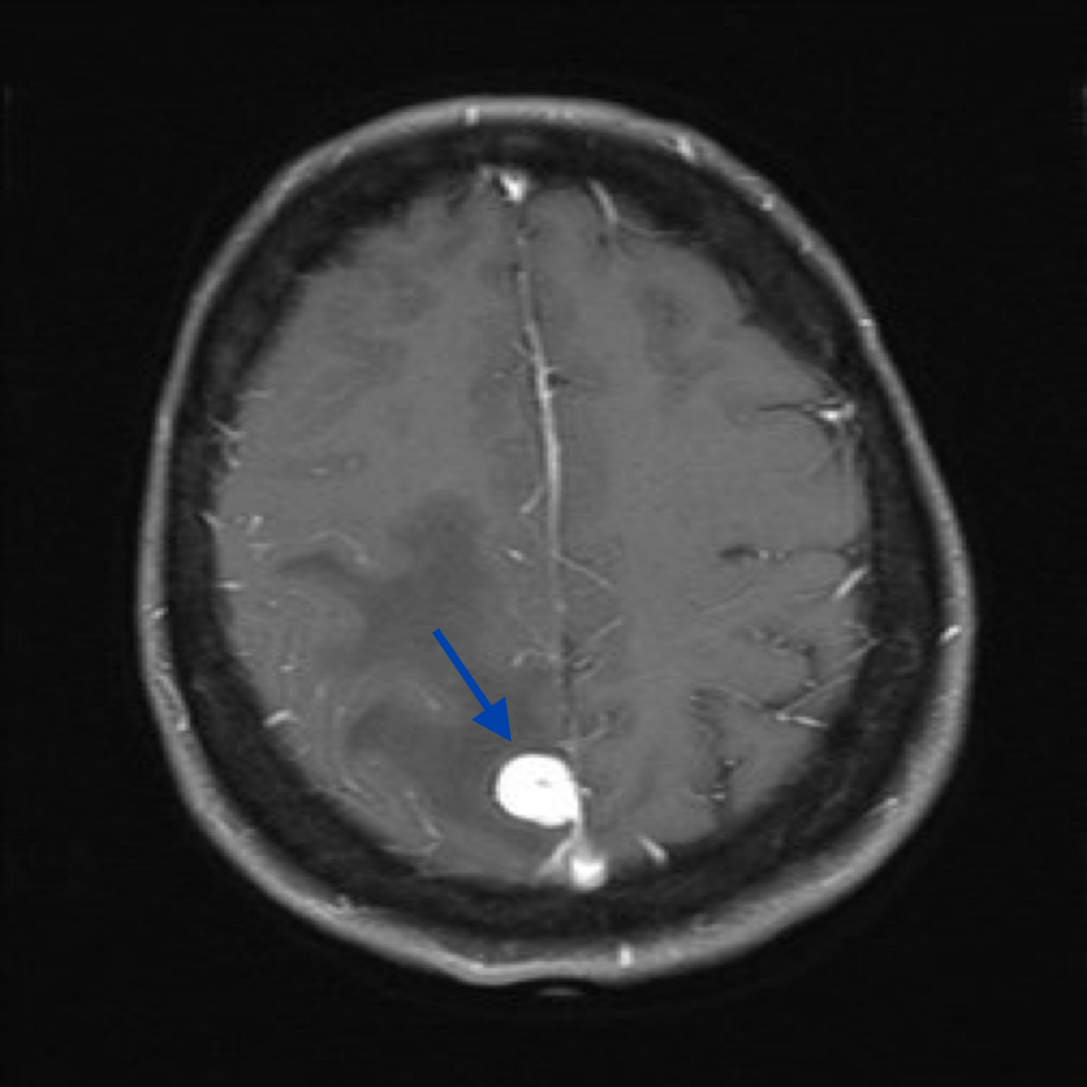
MRI, axial T1 post-gadolinium showing a well-demarcated extra-axial lesion with homogenous contrast enhancement and a small posterior dural tail MRI: magnetic resonance imaging
